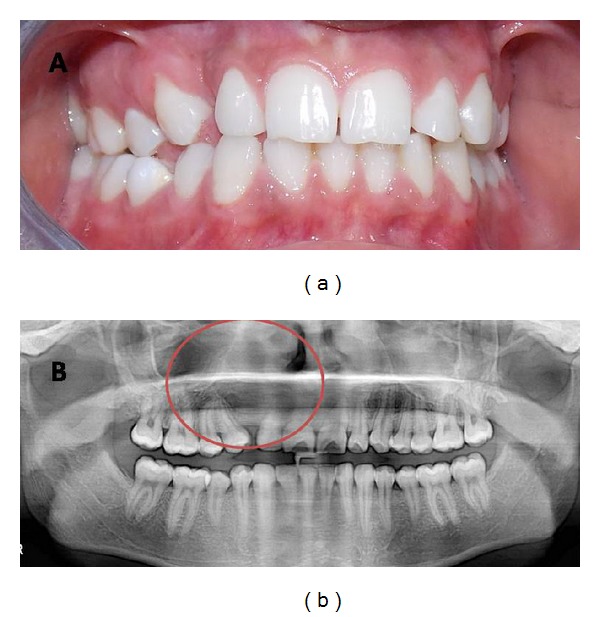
(a) Clinical presentation at 3-year follow-up. (b) Orthopantomograph taken at three- year follow-up.
